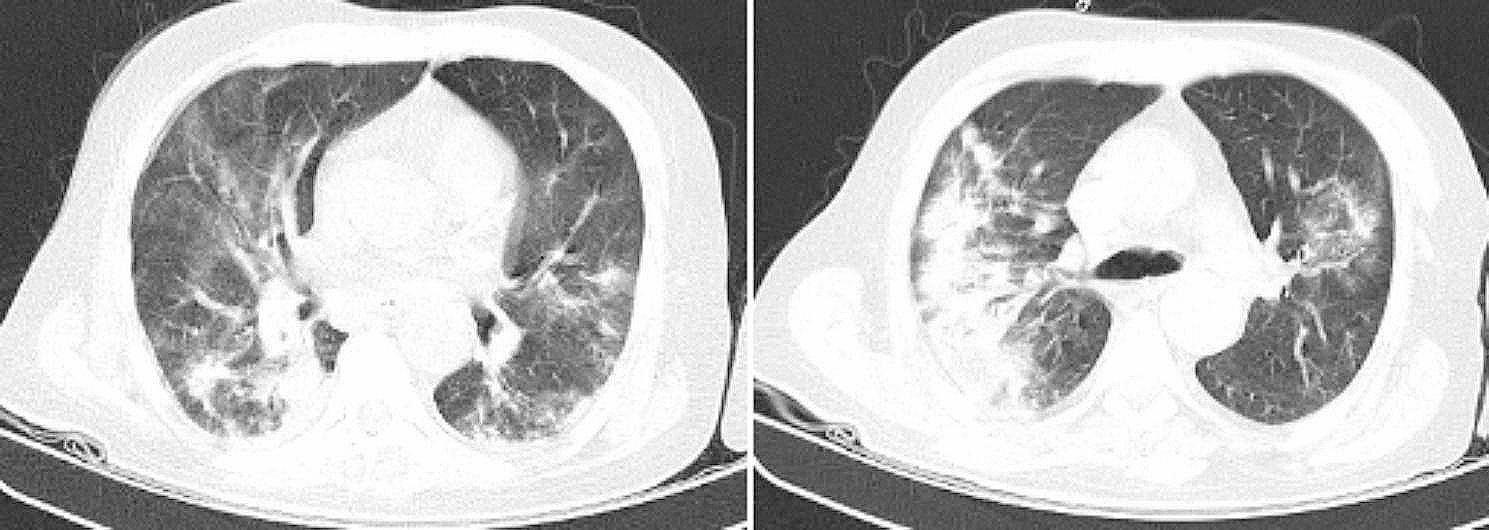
Chest CT scan shows multiple patchy ground glass opacities in favor of Covid-19 (Confirmed by PCR)
